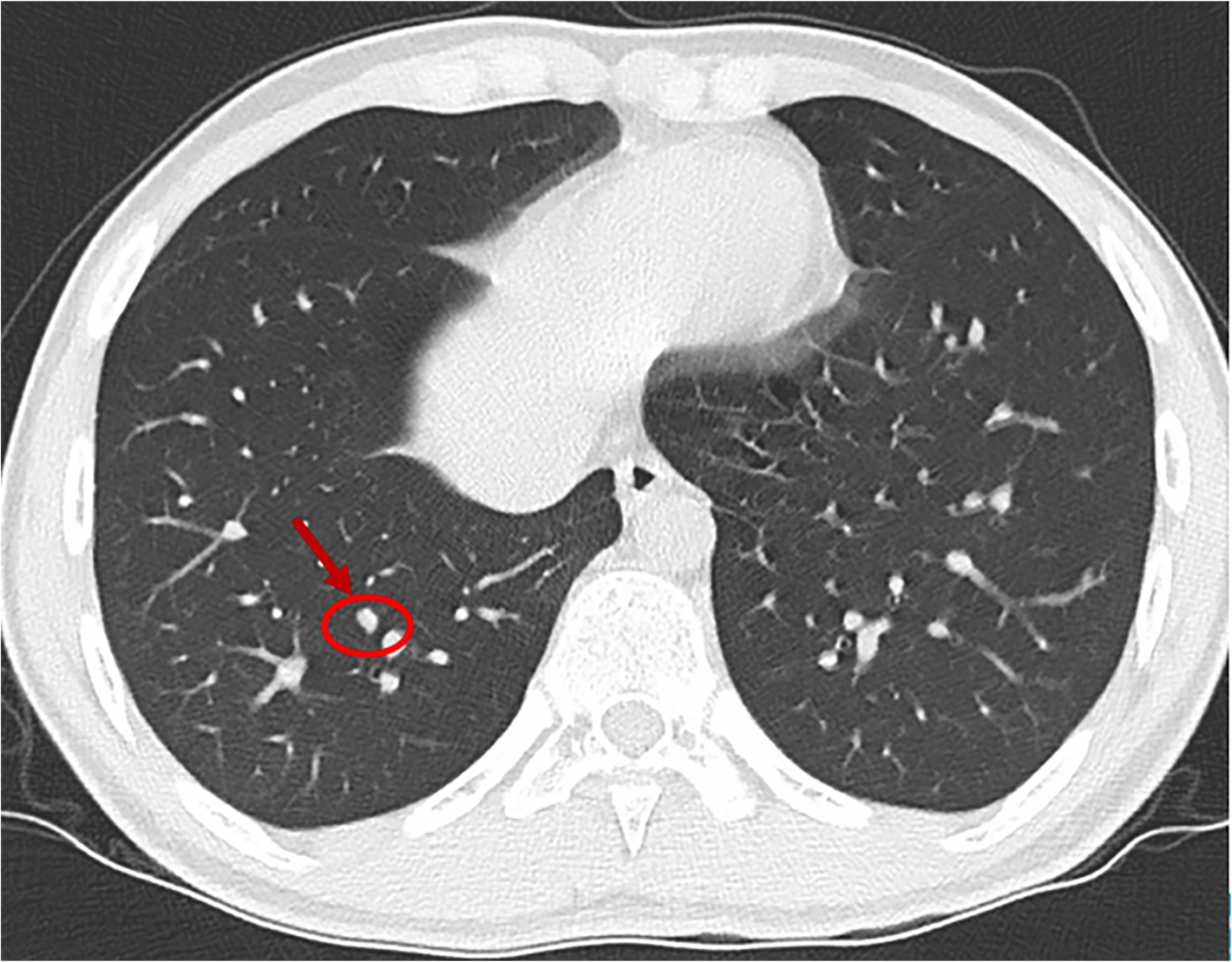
A nodule less than 5 mm missing on chest CT (red circle) for radiation (This image was taken before hypofractionated radiotherapy. CT = computed tomography)
